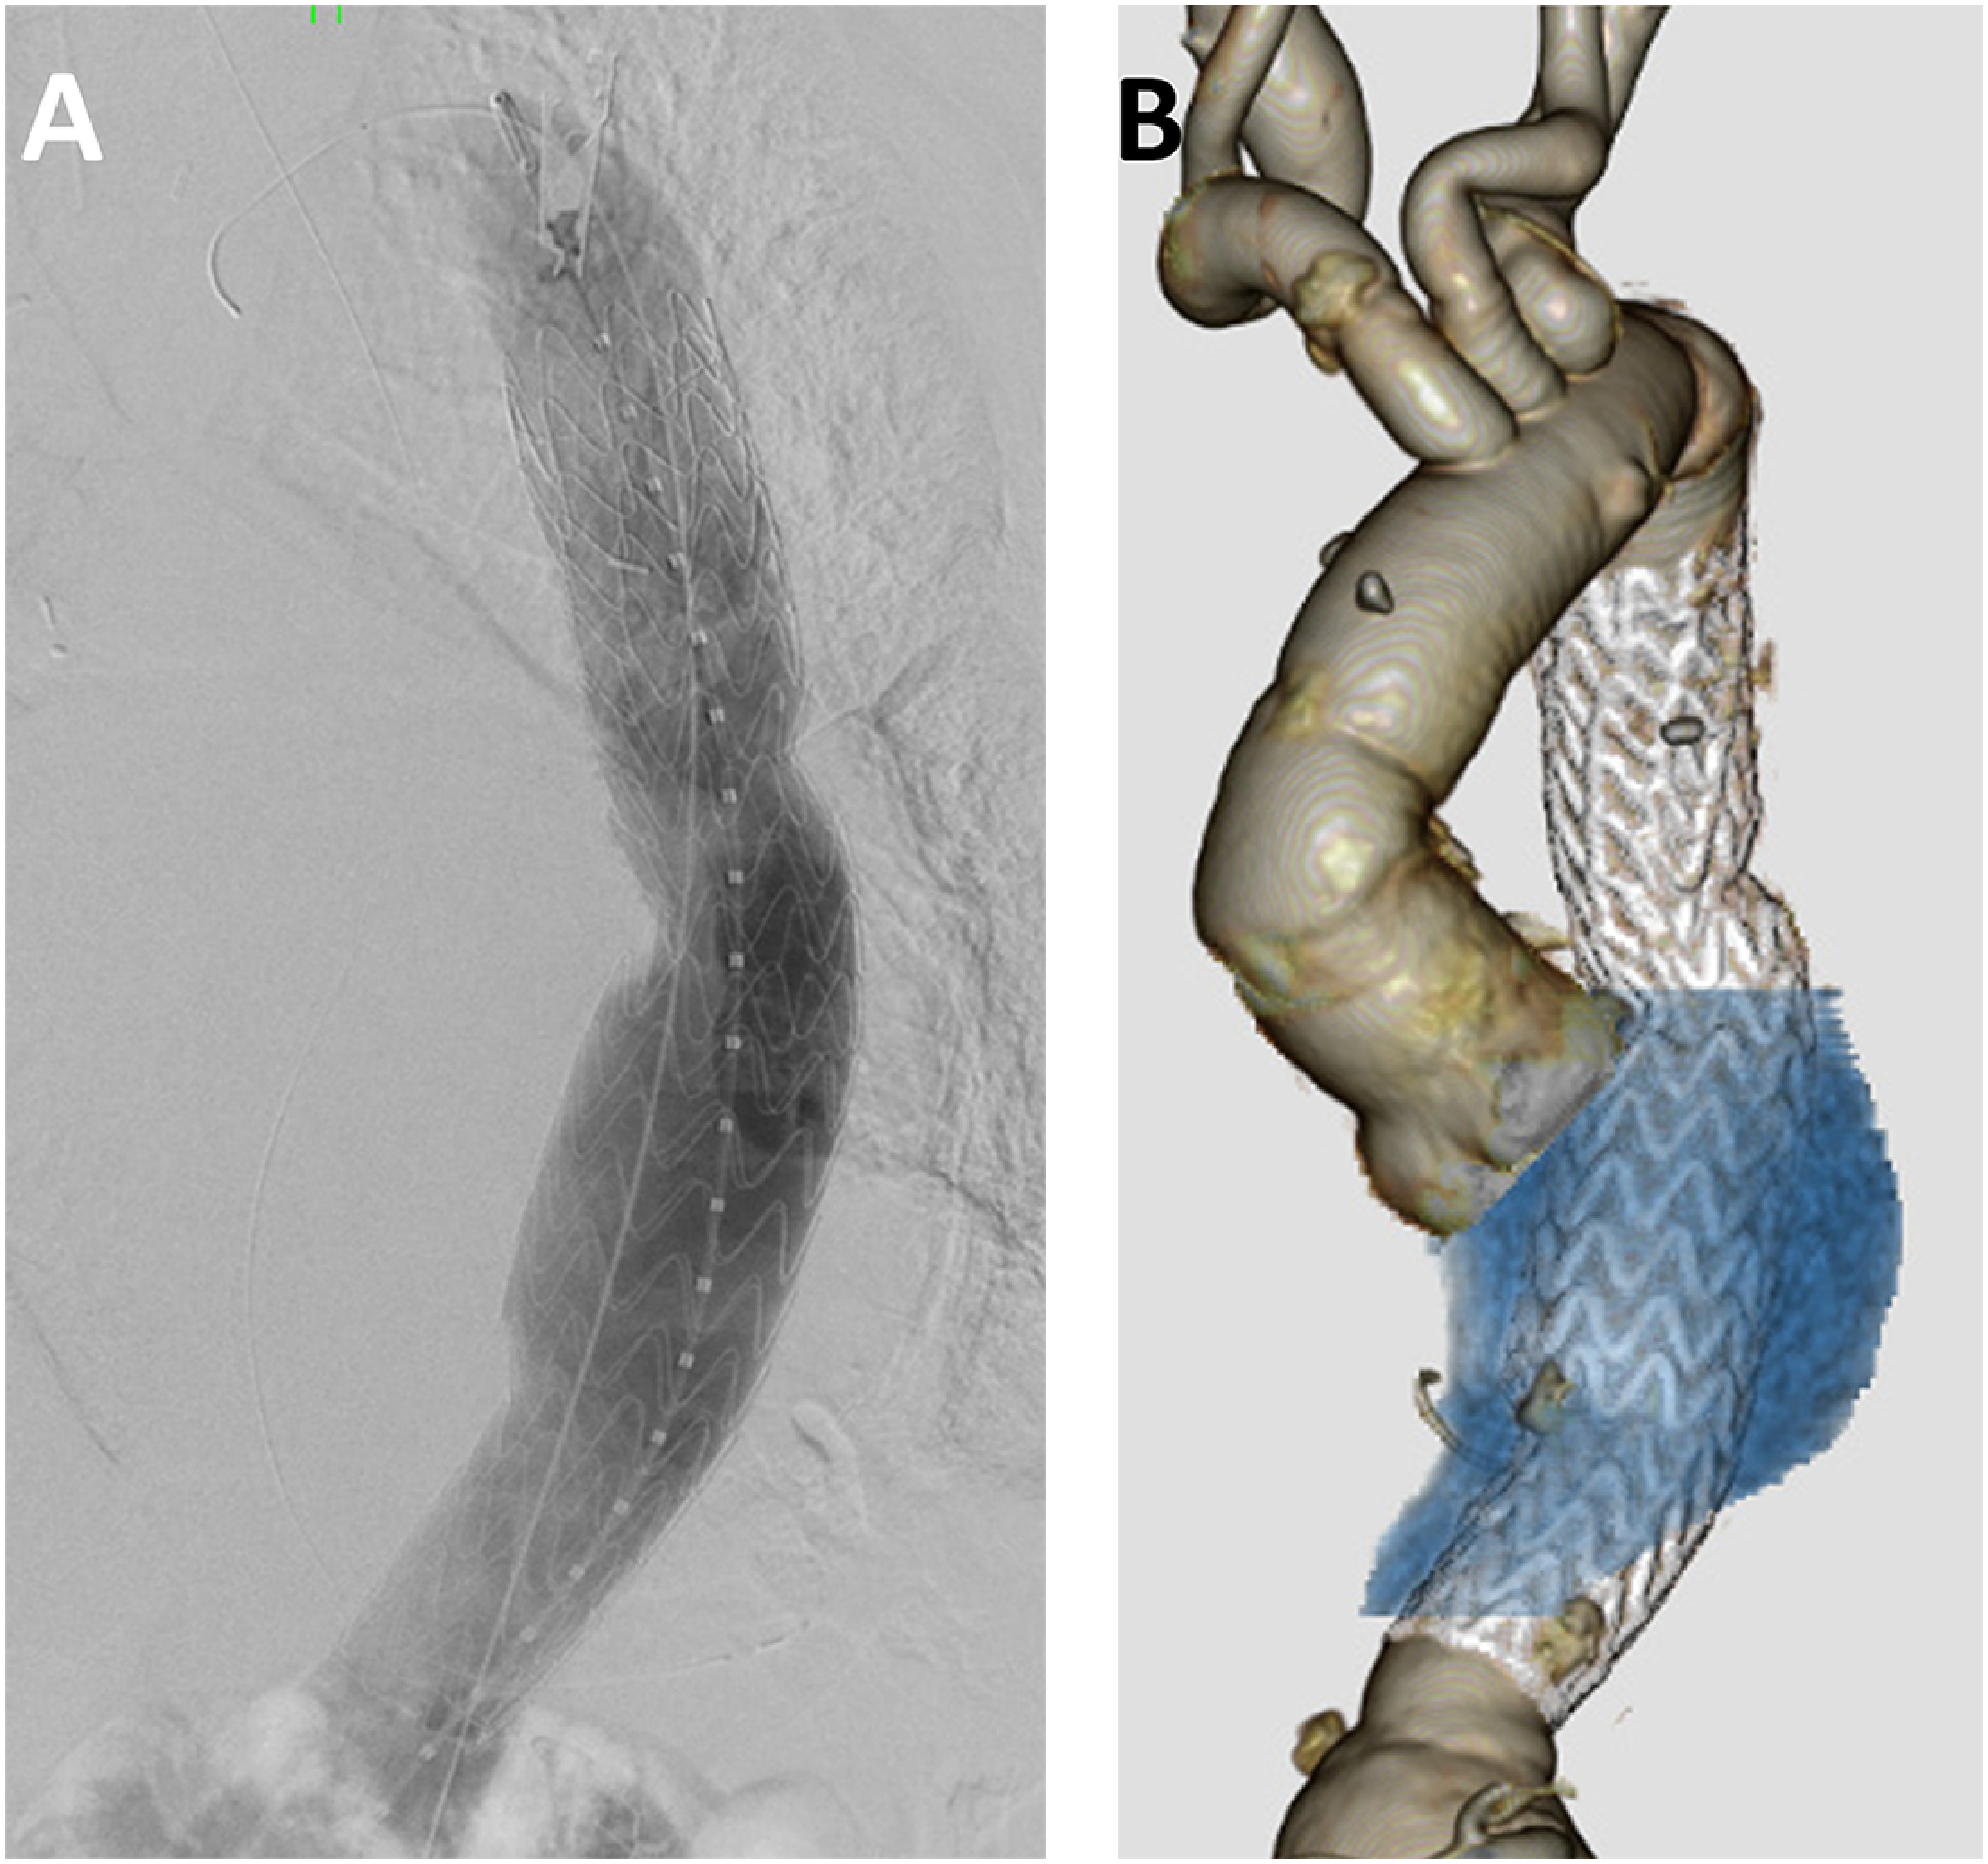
Fig. 2 Postoperative digital subtraction angiography (A) and enhanced computed tomography scan (B) were showing complete sealing of the intercostal patch aneurysm with stent graft of thoracic endovascular aortic repair and marked shrinking without endoleaks.
